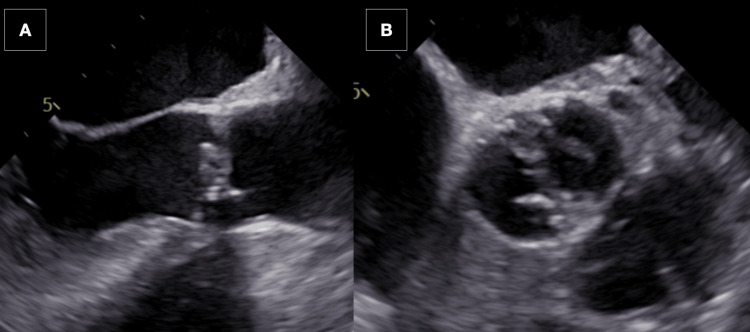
Preoperative transesophageal echography (A) Long-axis view of the aortic valve demonstrating restricted leaflet opening. (B) Short-axis view showing a bicuspid aortic valve with limited systolic excursion.
